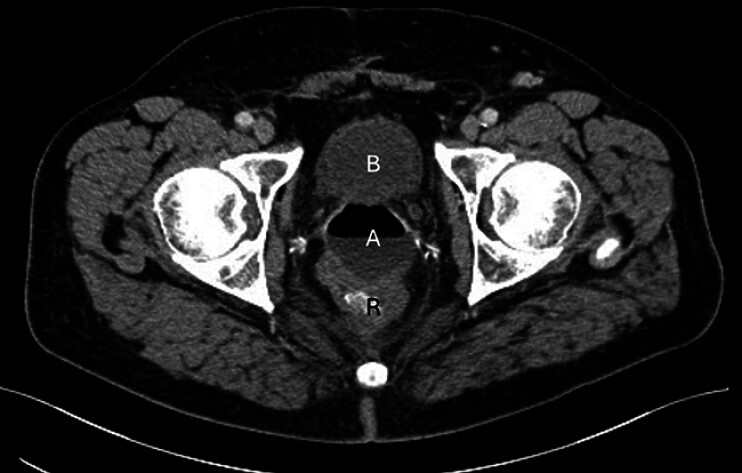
Computed tomography image of a perirectal abscess (A) with an air-fluid level, located
